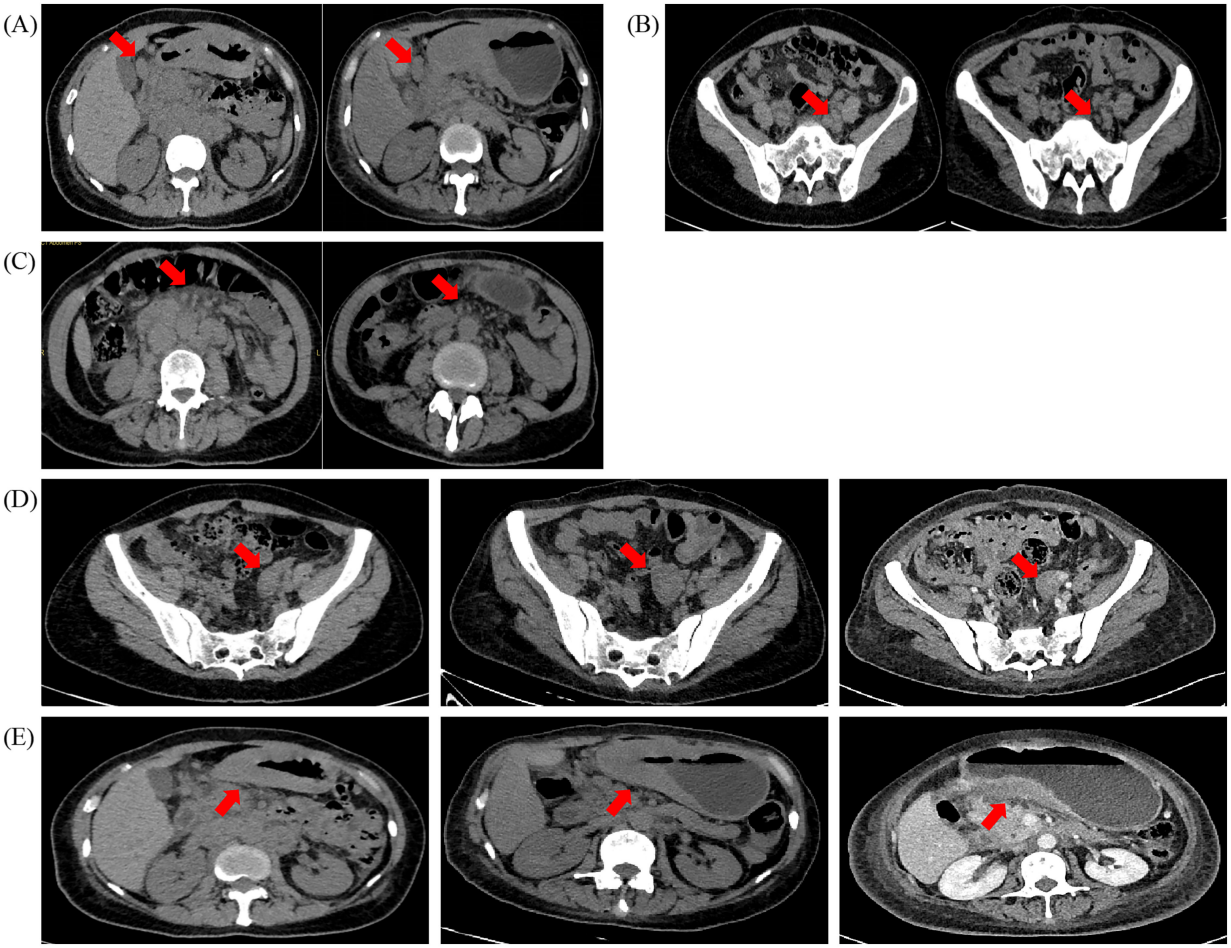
CT (Computed tomography) scans before (2023–08–03) and after (2023–11–20) (2024–03–04), the crizotinib treatments. (A) Metastatic lymph nodes around the gastric antrum and (B) pelvic metastatic lymph nodes were the two lesions that gradually shrunk significantly after the crizotinib treatments. (C) Omental metastatic lesions and (D) the pelvic adnexal mass shrunk significantly as well. E.The diffuse thickened gastric wall remained stable. All assessed lesions were indicated with red arrows.
